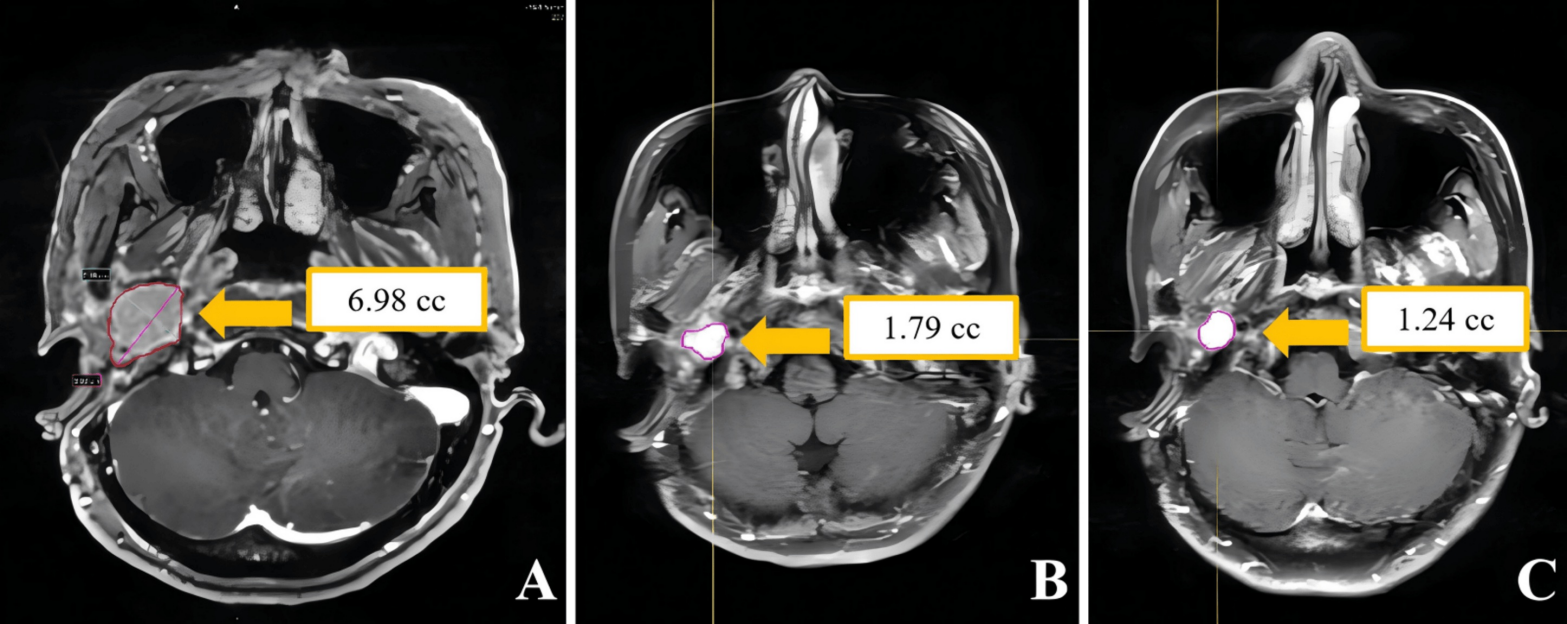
Contrast-enhanced MRI images of Case 1. (A) shows axial contrast-enhanced T1-weighted imaging with contouring of the tumor volume before radiosurgery; (B) shows the same sequence with tumor volume contouring one year post-ZAP-X radiosurgery; (C) shows the axial contrast-enhanced T1-weighted imaging with tumor volume contouring three years post-ZAP-X radiosurgery.
